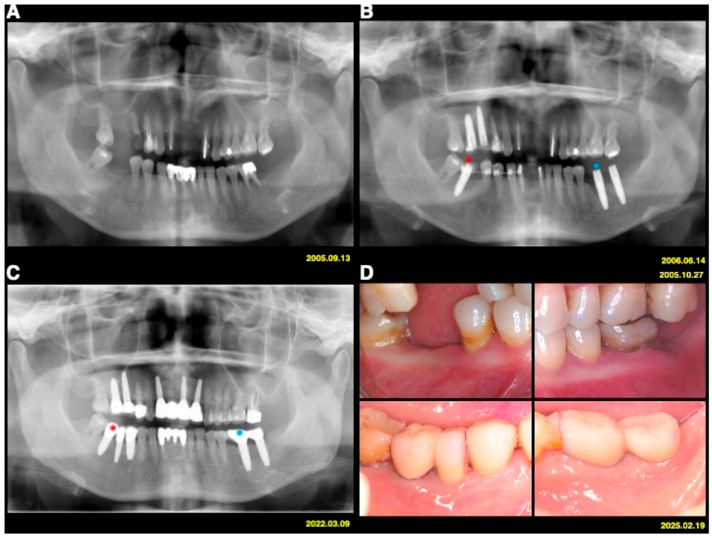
Case 1 (A) A 55-year-old female patient with Stage IV periodontitis. (B) Implants served as orthodontic anchorage (test; red stars) and for prosthetic rehabilitation only (controls; blue stars). (C) Radiographs in 2022 showed no periodontal or peri-implant bone loss. (D) Clinical findings at 20 years post-treatment demonstrated stable and healthy periodontal and peri-implant tissues.
